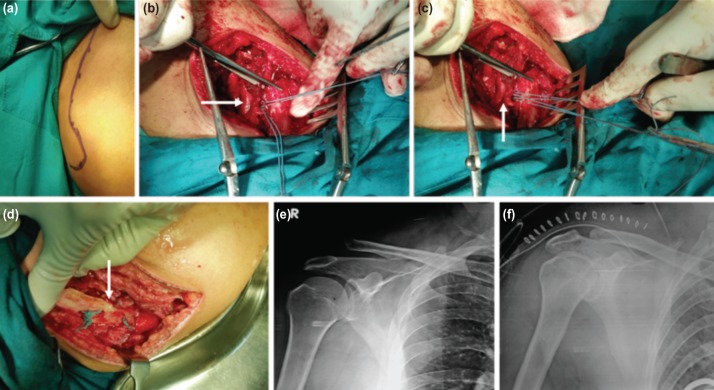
(a) Marking through the clavicle and acromian in longitudinal direction. (b,c) Resection of lateral end of clavicle and tying of clavicle in reduced position with nonabsorbable Ethibond suture passed through undersurface of coracoid process. (d) Two separate knots on lateral and medial aspect of clavicle for secure of graft and maintainance of clavicle in reduced position respectively. (e) Preoperative radiograph showing the type V AC joint dislocation. (f) Postoperative radiograph showing reduced clavicle at the level of acromion.
